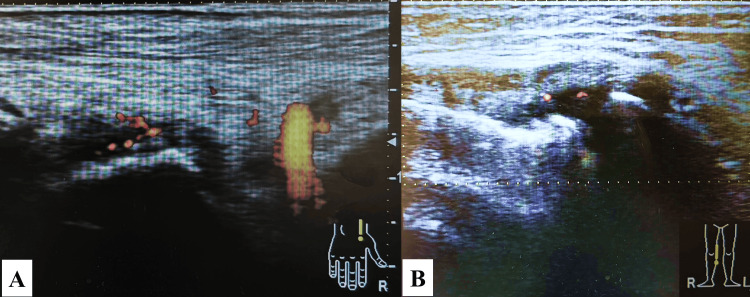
Ultrasonography of right wrist joint (A) and right knee joint (B) Ultrasonography shows synovial thickening with an active Doppler signal (color) indicating synovial inflammation.
